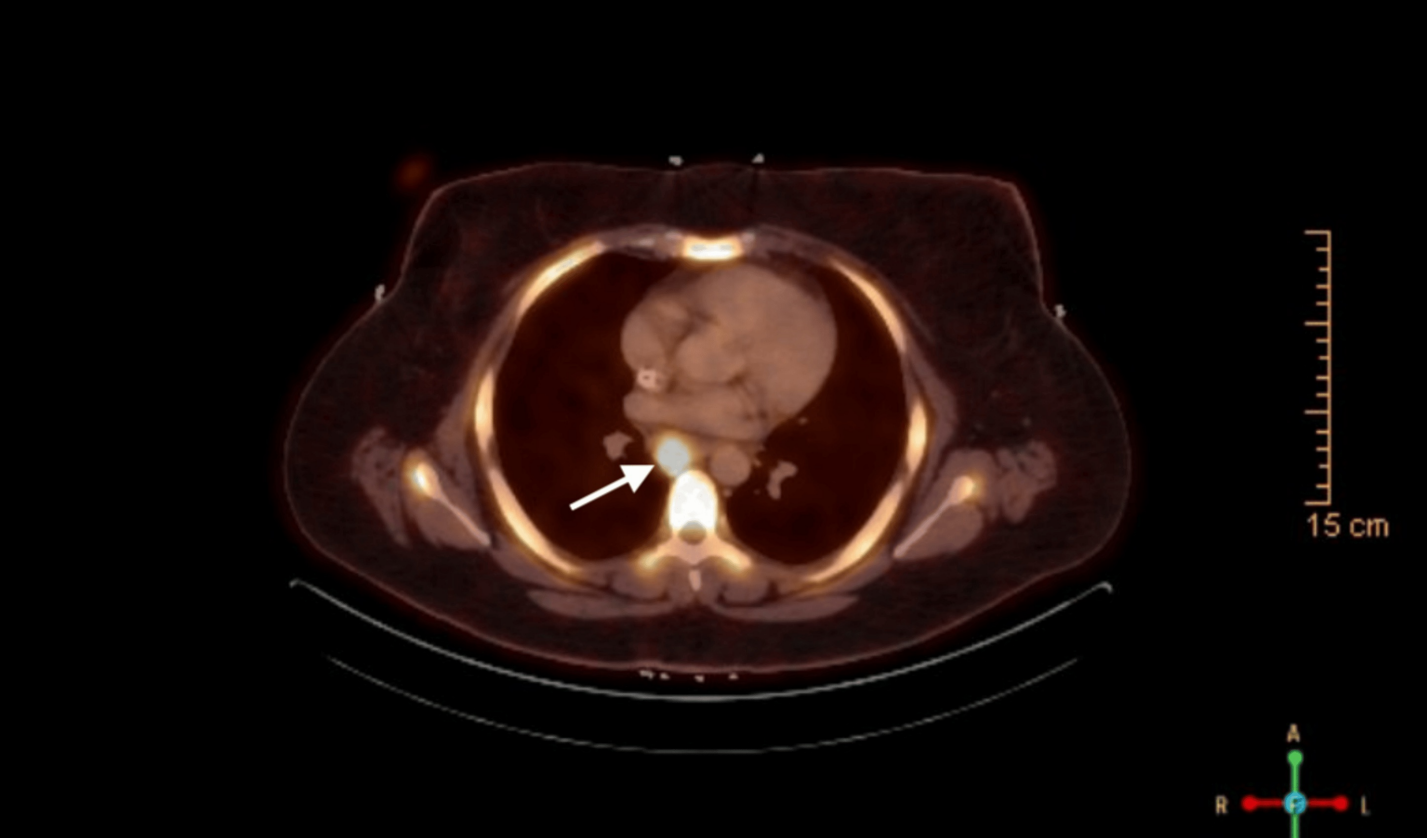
PET-CT scan demonstrating suboptimal response to therapy The PET-CT scan from January 2024 shows persistent FDG uptake in the mediastinal lymph nodes, with a reduced SUVmax of 19 in comparison to the baseline SUVmax of 27. The reduction in lymph node size is limited to <30%. This indicates a suboptimal therapeutic response. These findings suggest residual disease activity and inadequate metabolic response to the administered chemotherapy regimen. PET-CT: positron emission tomography-computed tomography; FDG: fluorodeoxyglucose; SUVmax: maximum standardized uptake value
